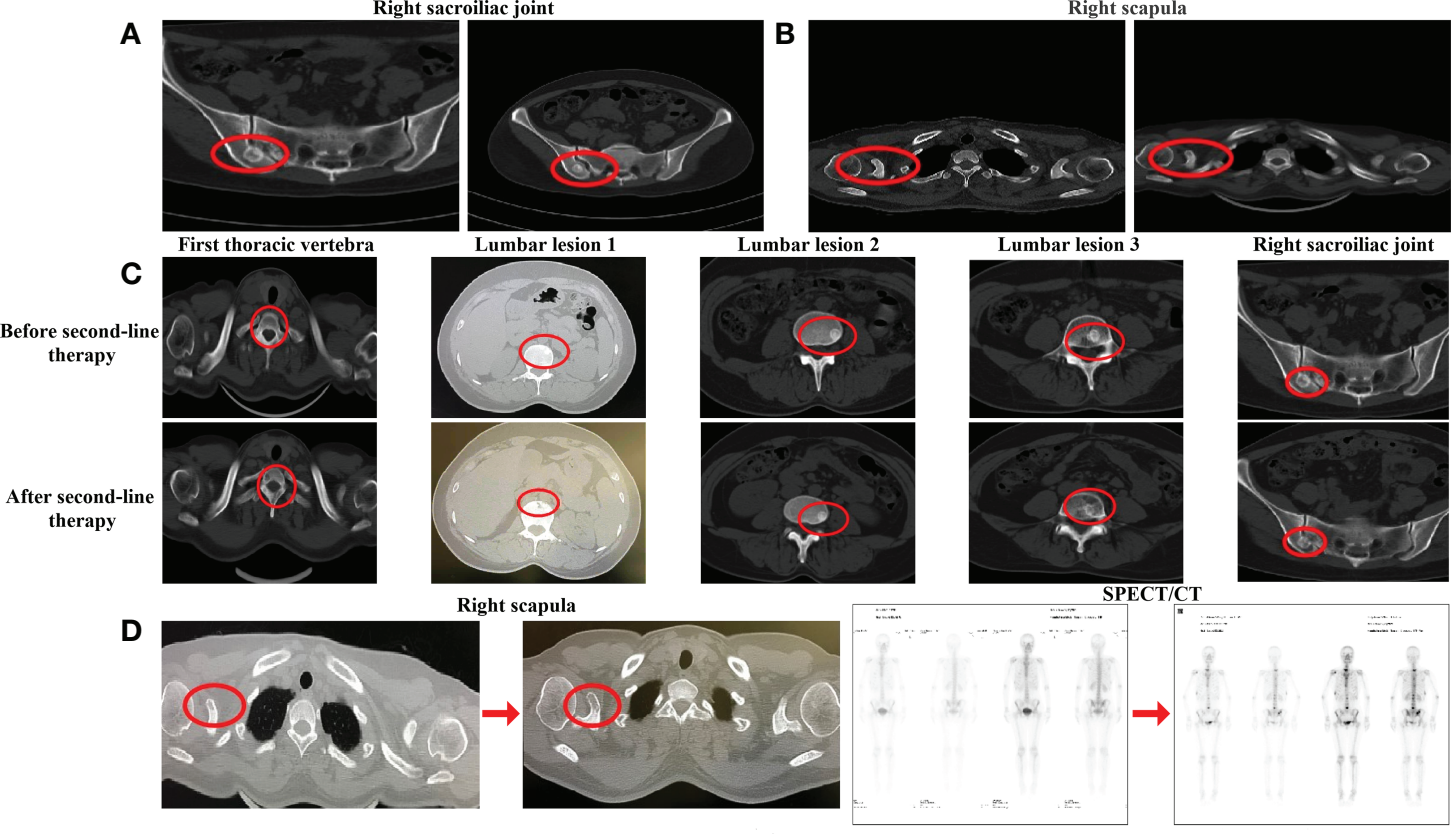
Evaluation after second-line chemotherapy. (A) CT evaluation of the right sacroiliac joint before chemotherapy and after cycles in December 2020. (B) CT evaluation of the right scapula before chemotherapy and after chemotherapy in May 2021 (C) CT evaluation of the bone lesion after second-line therapy in July 2021, (D) CT evaluation of the right scapula and SPECT evaluation after second-line therapy in July 2021.
